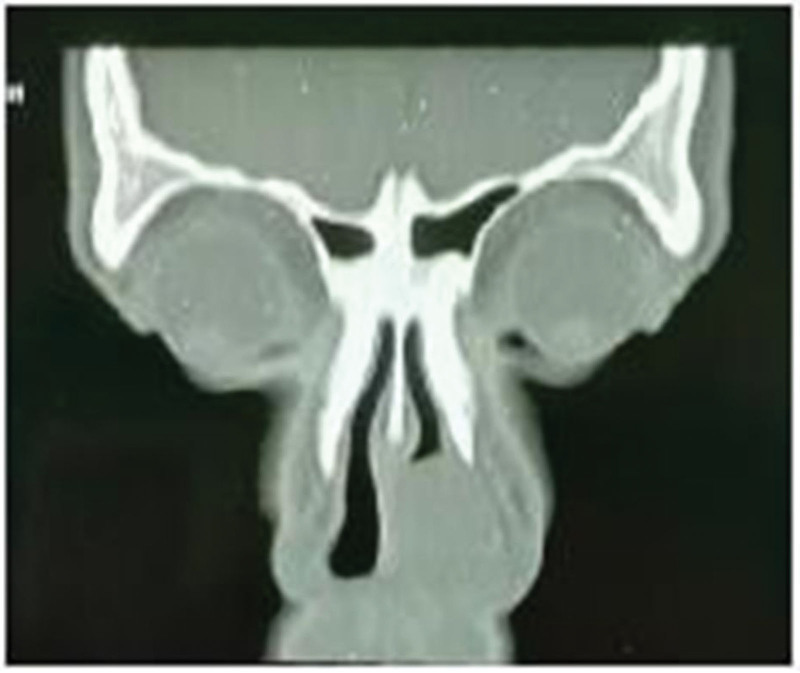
CT of the sinuses showed a left nasal vestibule mass.
